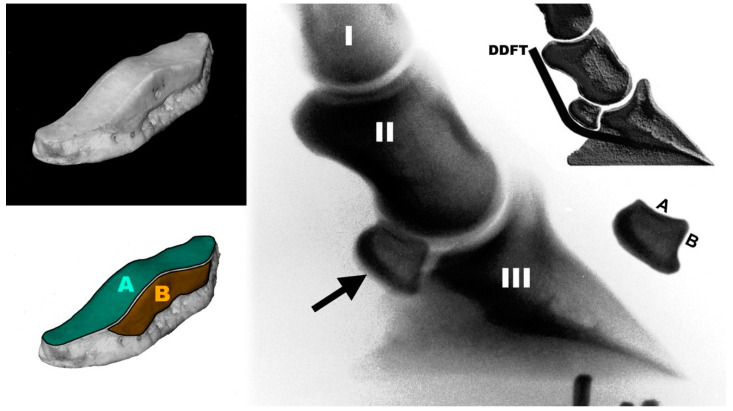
Navicular bone, iso-view, and medio-lateral radiograph (A: joint surface for the middle phalanx, B: joint surface for the hoof bone; I: proximal phalanx, II: middle phalanx, III: hoof (coffin) bone; arrow: navicular bone; DDFT: deep digital flexor tendon).
